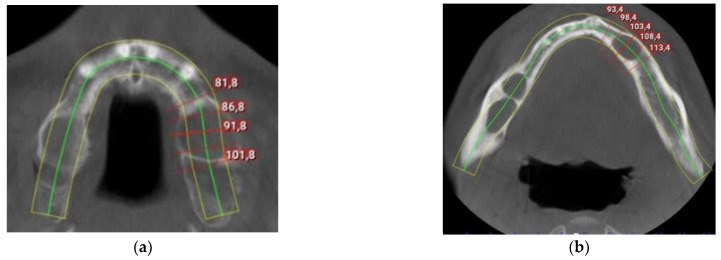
Preoperative CBCT scan: (a) maxillar keratocyst in the left upper region; (b) keratocysts in the mandible.
